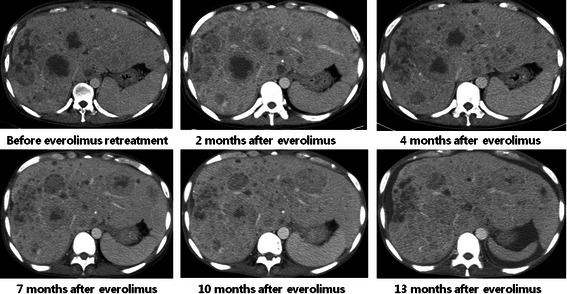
These serial CT scans demonstrate the efficacy of re-treatment with everolimus 10 mg/d. The liver metastases remained stable during the period of re-treatment with everolimus for over 2 years.
